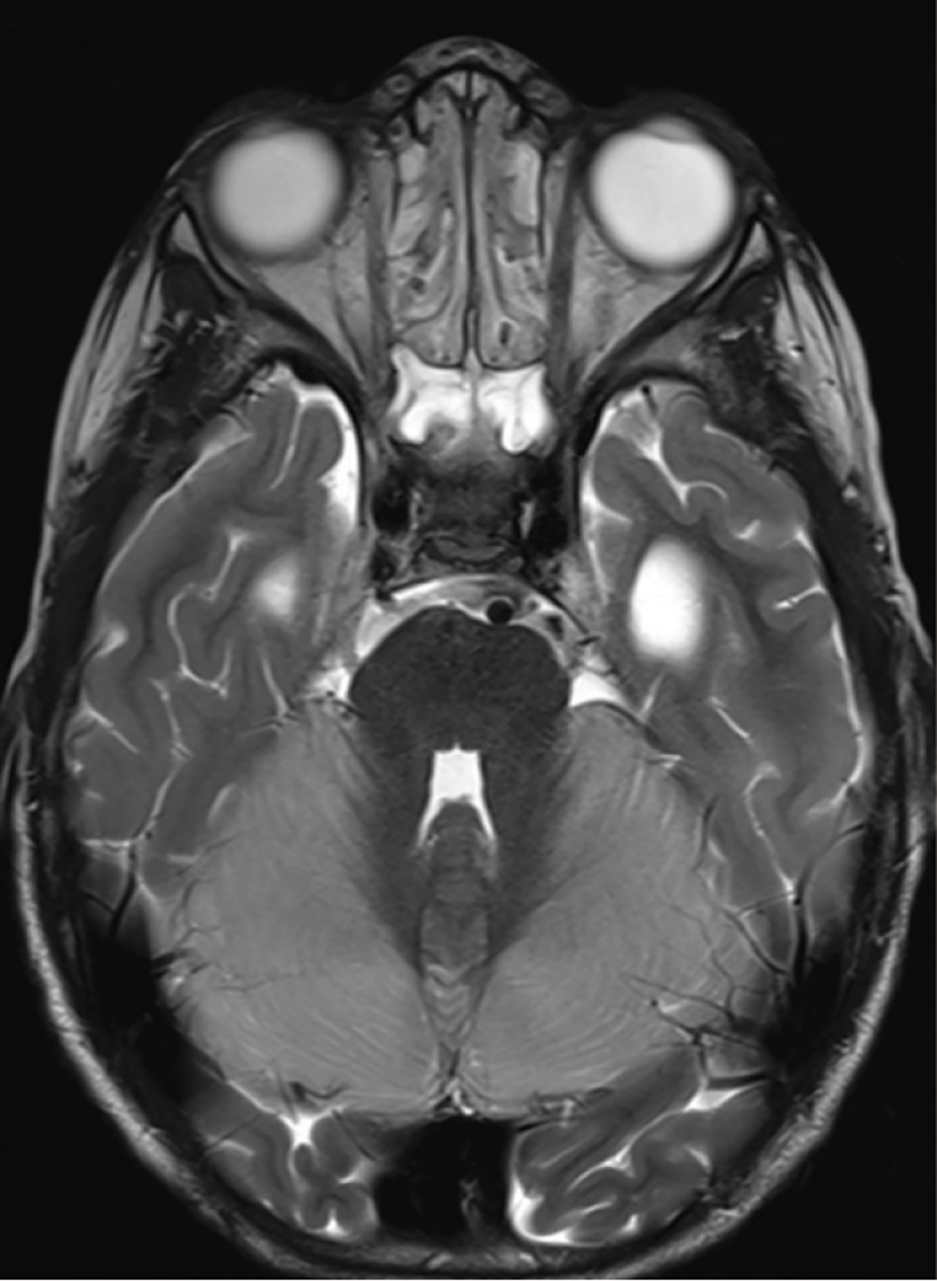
Axial T2‑weighted MRI shows a hyperintense signal in both cerebellar hemispheres and the brainstem, indicative of edema.
